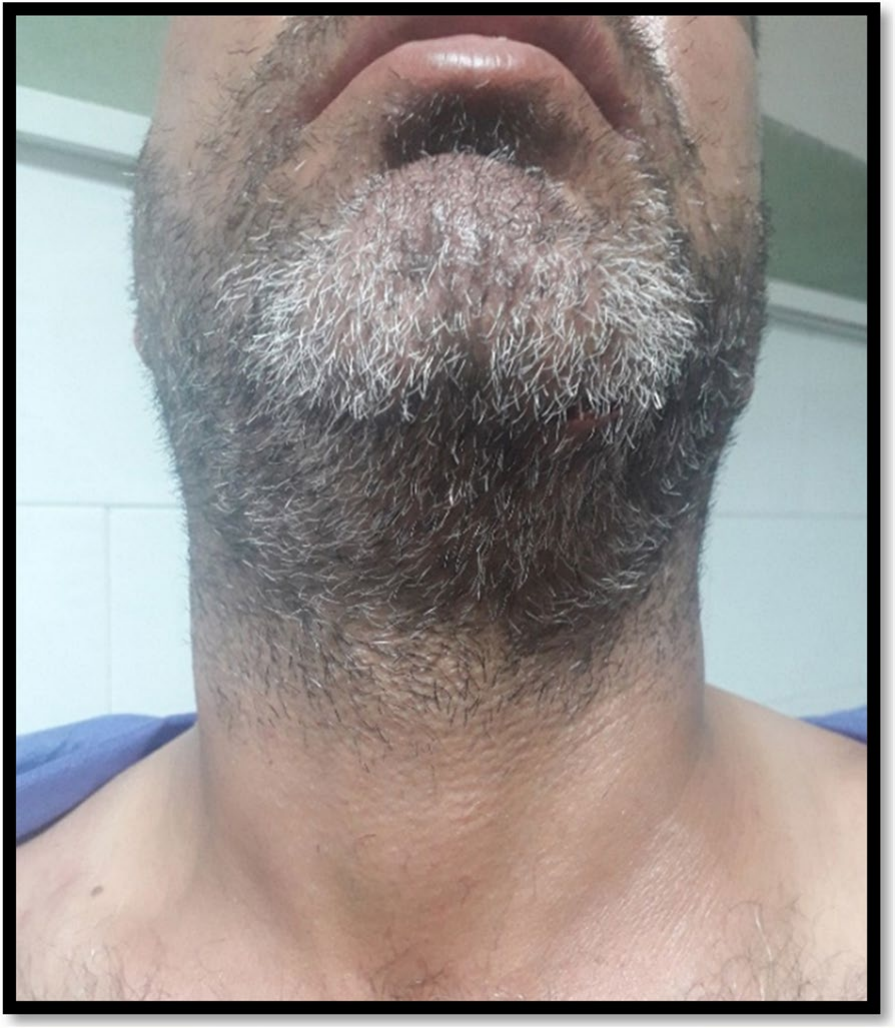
No ecchymosis, bruising, hematoma, and external bleeding in the trauma site at the neckD: The patient was alert with the Glasgow Coma Scale/Score (GCS) of 15/15, and there was no cervical spine pain and tenderness, while the cervical collar was fixed.E: The patient was exposed while kept warm, and there were no other findings in the physical examinations.There was no abnormality in the simple radiography of the cervical spine. Color Doppler sonography (CDS) of the carotid and vertebral arteries and jugular vein showed normal flow velocity and spectral waveforms in the common carotid artery (CCA), internal carotid artery (ICA), external carotid artery (ECA), and vertebral arteries, and there was normal flow in both internal jugular veins.While the patient’s hoarseness and dyspnea got worse with time, neck and chest CT scans were performed to rule out laryngeal and other chest trauma. The CT scans showed no pathology in the chest but comminuted fracture of the left anterior arch of the cricoid cartilage with left-sided mucosal thickening, inflammation, and edema which was extended to the glottis, causing a narrowing of the airway (transverse inner diameter of the cricoid = 3.7 mm) (Fig. 3).
